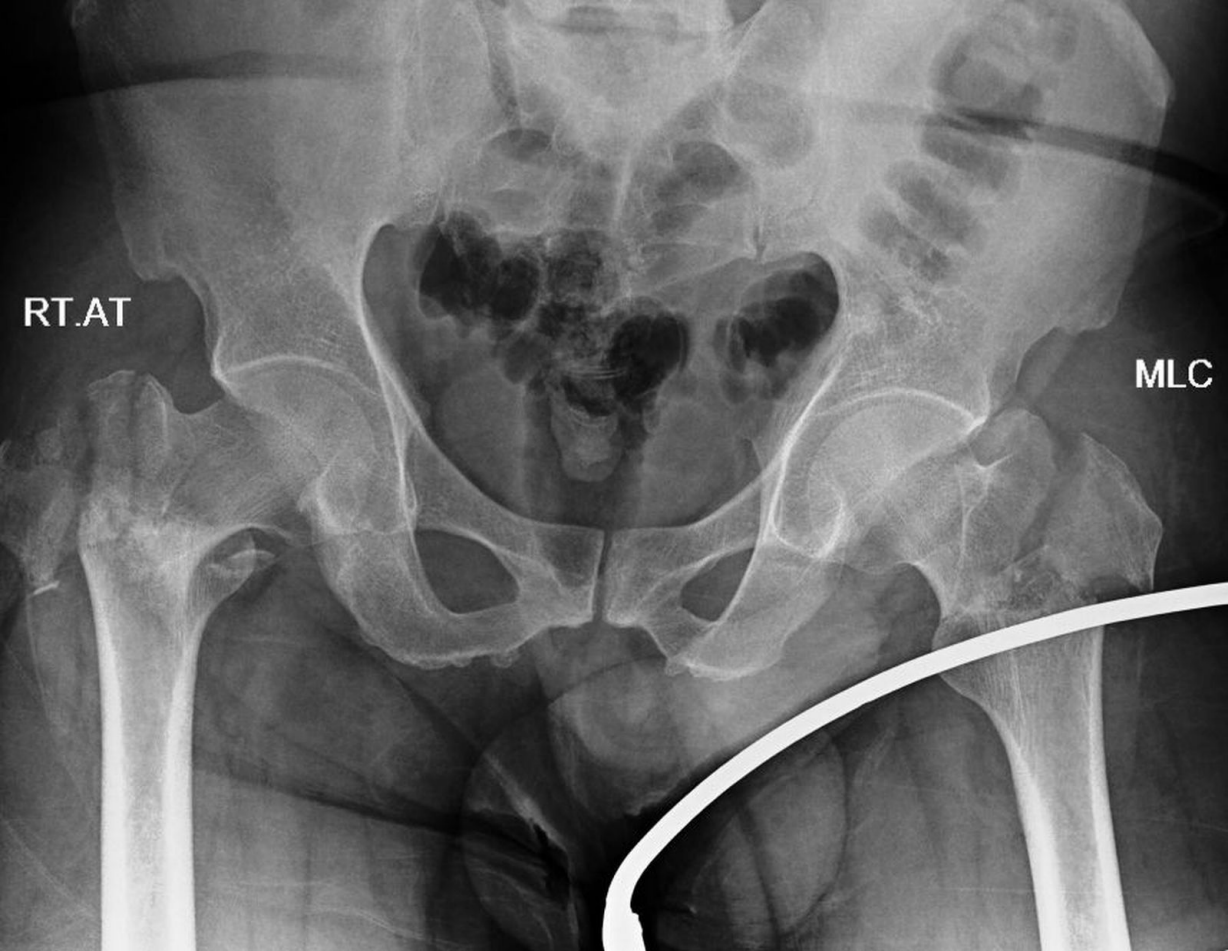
X-ray of the pelvis showing bilateral comminuted intertrochanteric fractures
